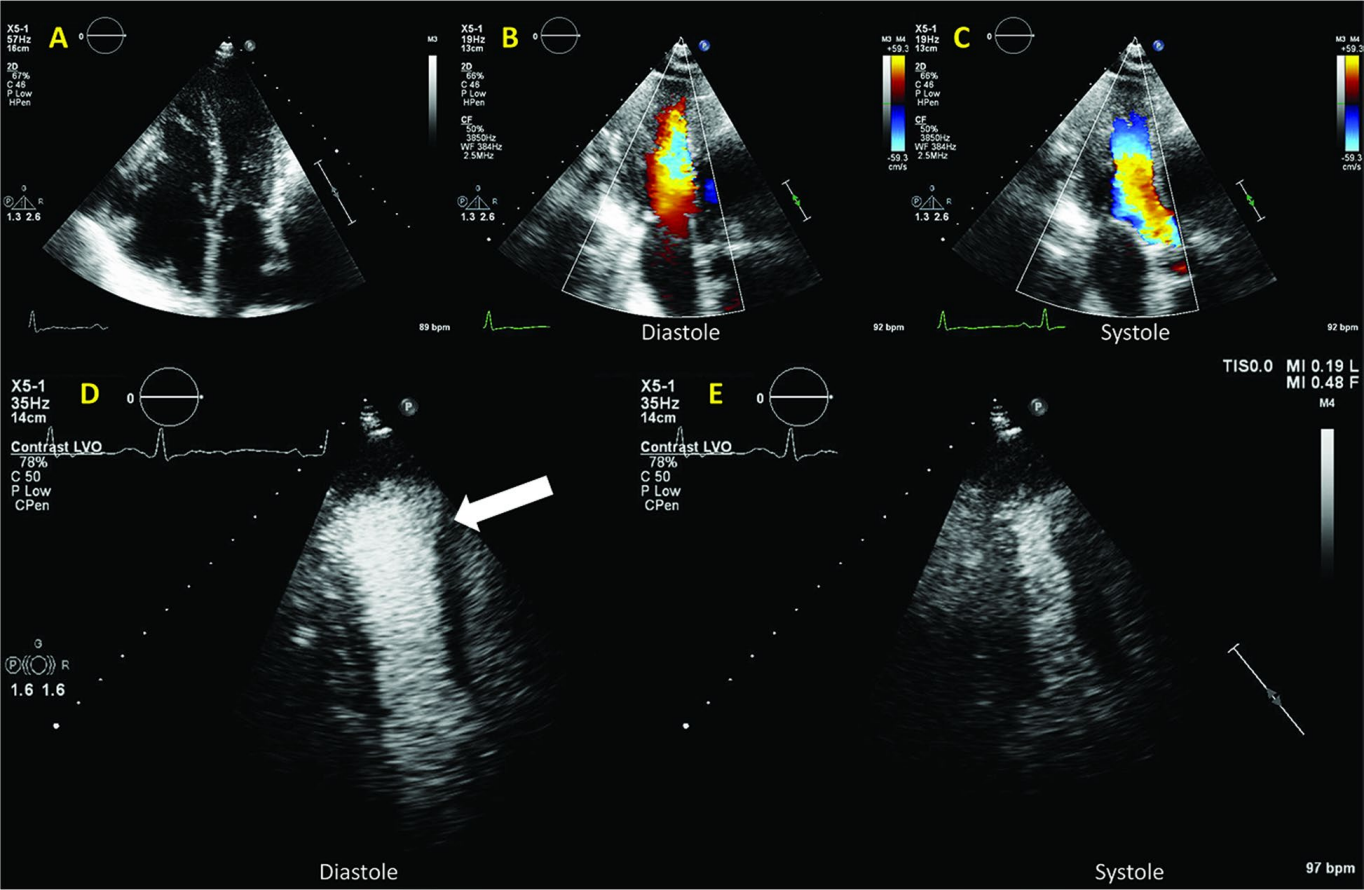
Patient 2. A: 2D echocardiography: LV compressed dilated RV, but with normal systolic function; signs of pressure and volume overload; B, C: Color Doppler of the LV in diastole (B) and systole (C). D, E: clinical contrast in diastole (D) and systole (E). The LV in diastole (arrow) forms a diastolic dilatation of a rather hyperkinetic apex; there is also a near-collapse of the mid-LV cavity
